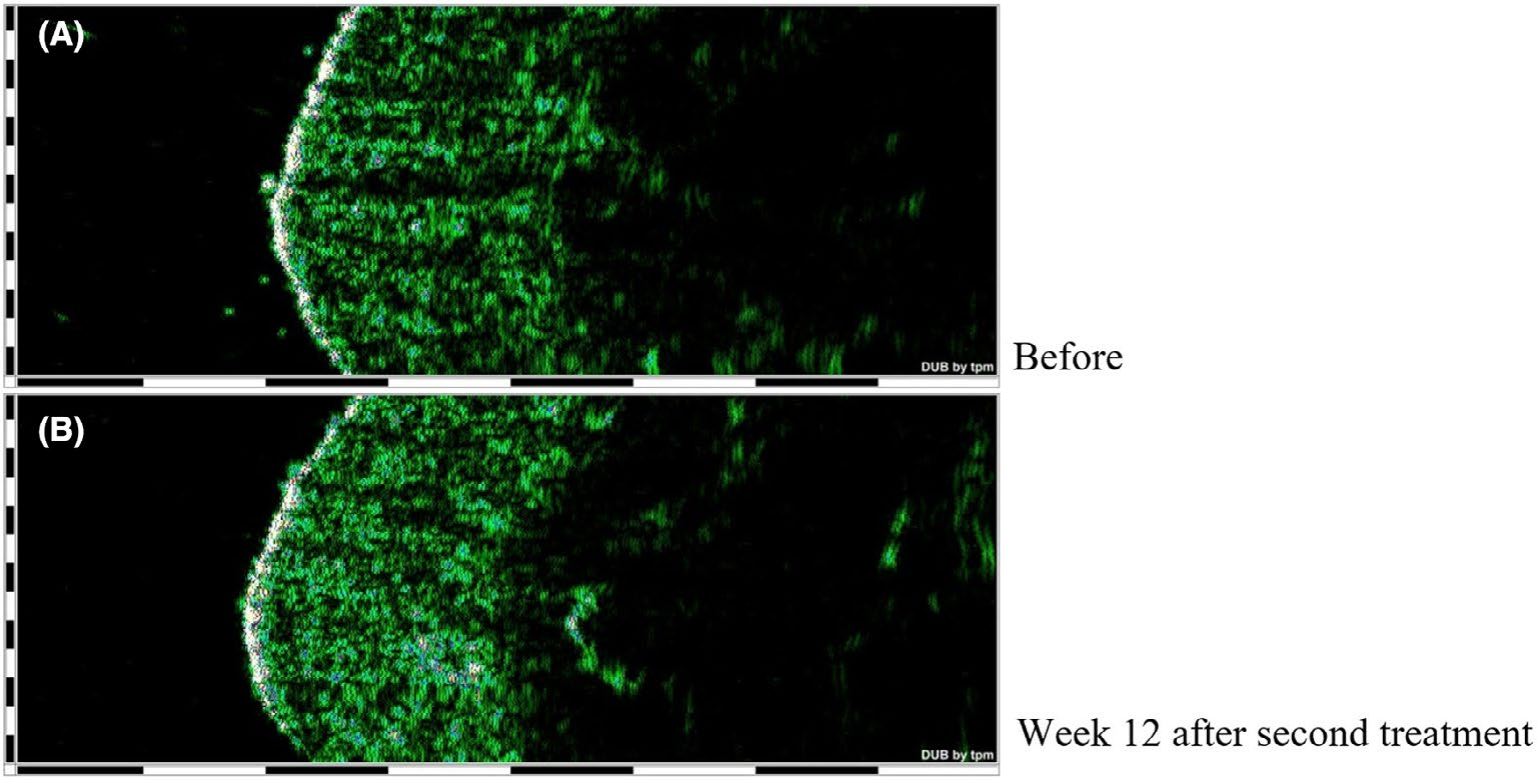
Dermis thickness (unit: μm) and density (unit: Arbitrary) improvement in a 44‐year‐old man, evaluated by 22 MHz ultrasound probe (A: Before treatment/B: Week 12 after second treatment).
